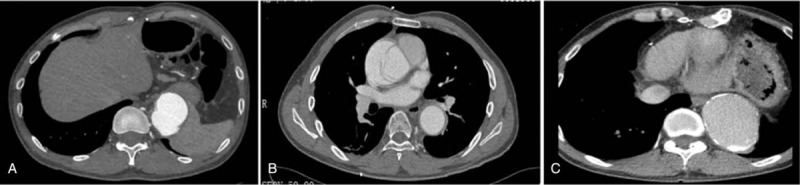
A: The first CTA (2005) revealed an abdominal aortic aneurysm dissection. B: The second CTA (2012) revealed a sign of Stanford type A aortic dissection. C: The third CTA revealed an aneurysmal dilatation of the descending aorta.
